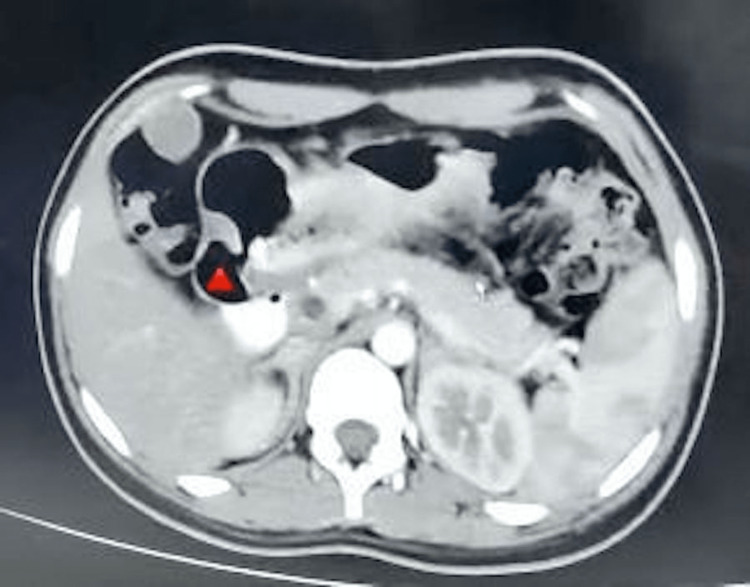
Dilated Bowel Loops, CT image Dilated bowel loops with clumping of bowel loops involving proximal jejunal loops with normal distal flow contrast into distal loops
